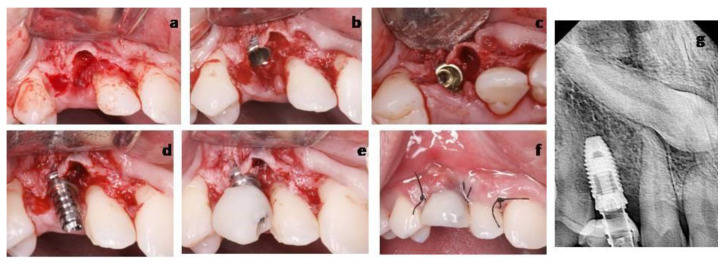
Implant and provisional crown placement (Case 2). (a) Abuccal full-thickness flap was raised until the buccal alveolar crest was exposed. (b) Inserted implant: Apically from the cementoenamel junction of adjacent teeth. (c) Implant occlusal view. (d) Implant abutment. (e) Provisional screw resin crown. (f) Flap was adapted and sutured. (g) Periapical radiograph.
